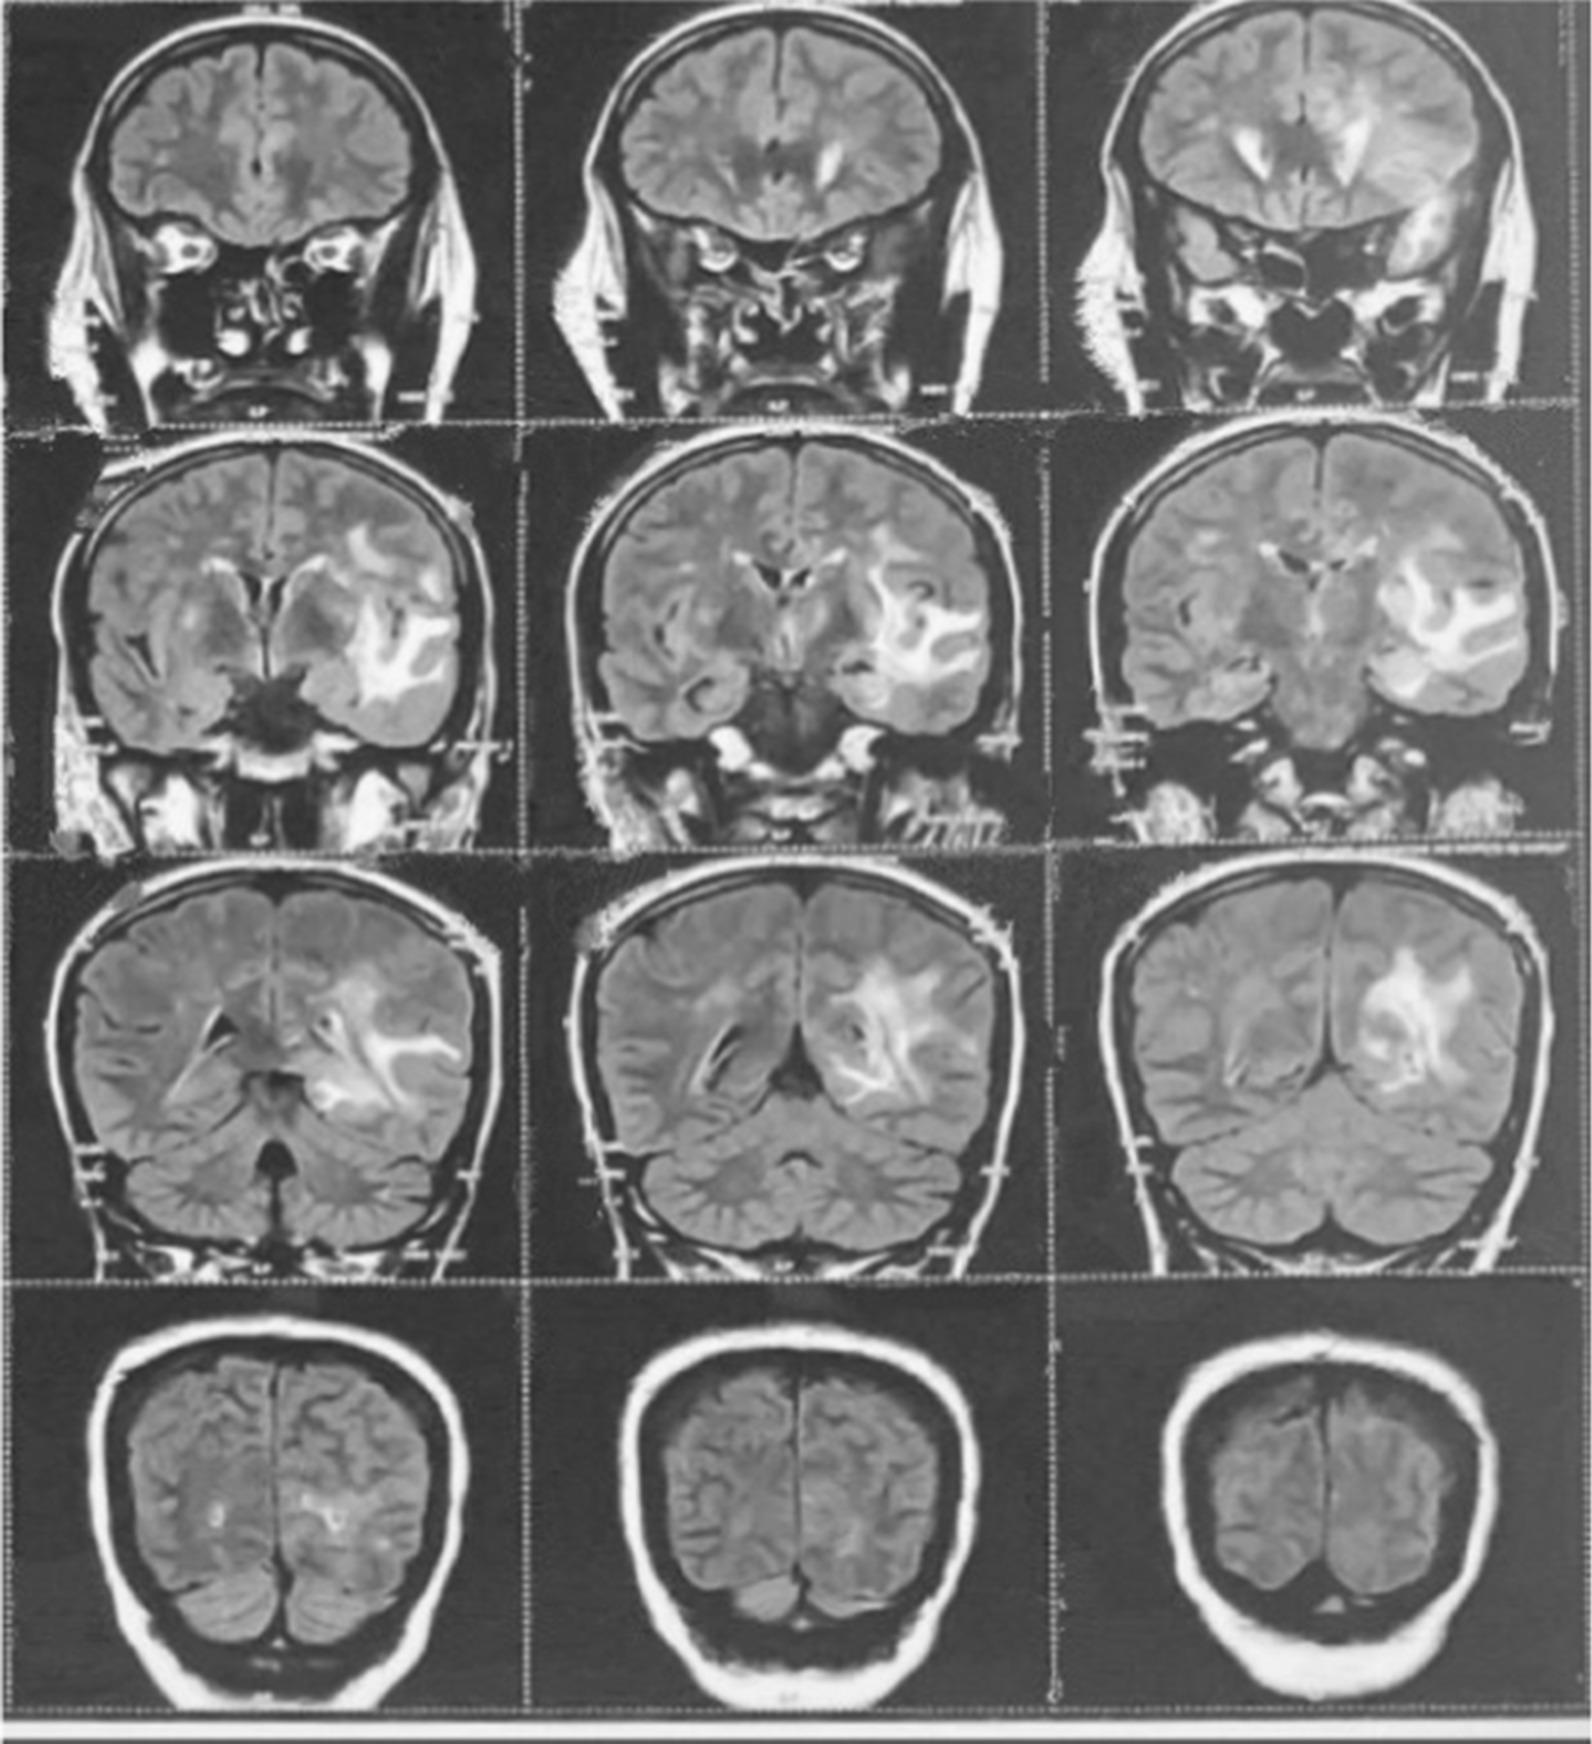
Magnetic resonance imaging (MRI). Brain with features suggestive of Atypical PRES
